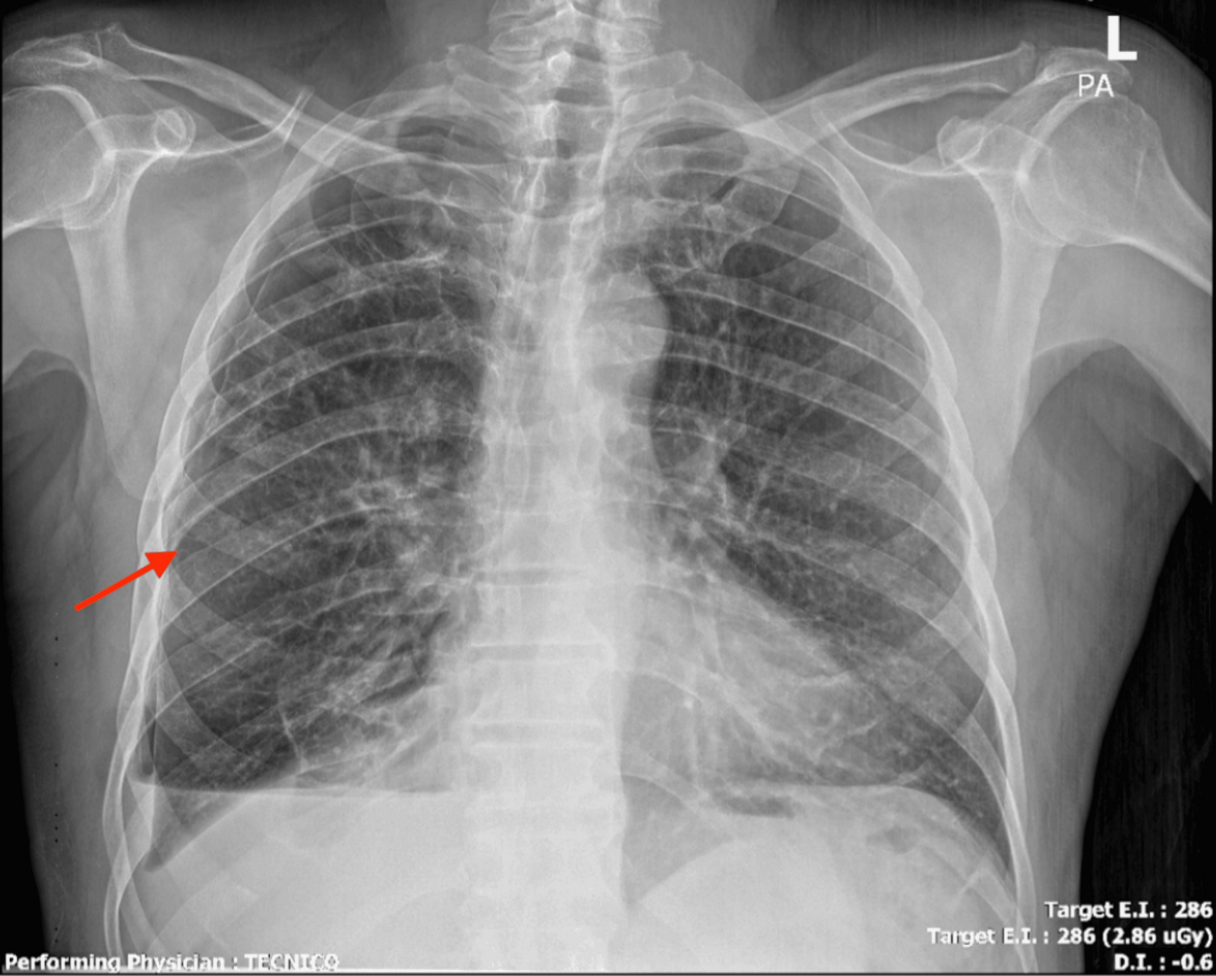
Post-ABPP chest radiograph showing lung re-expansion and resolution of pneumothorax. ABPP: Autologous blood patch pleurodesis
